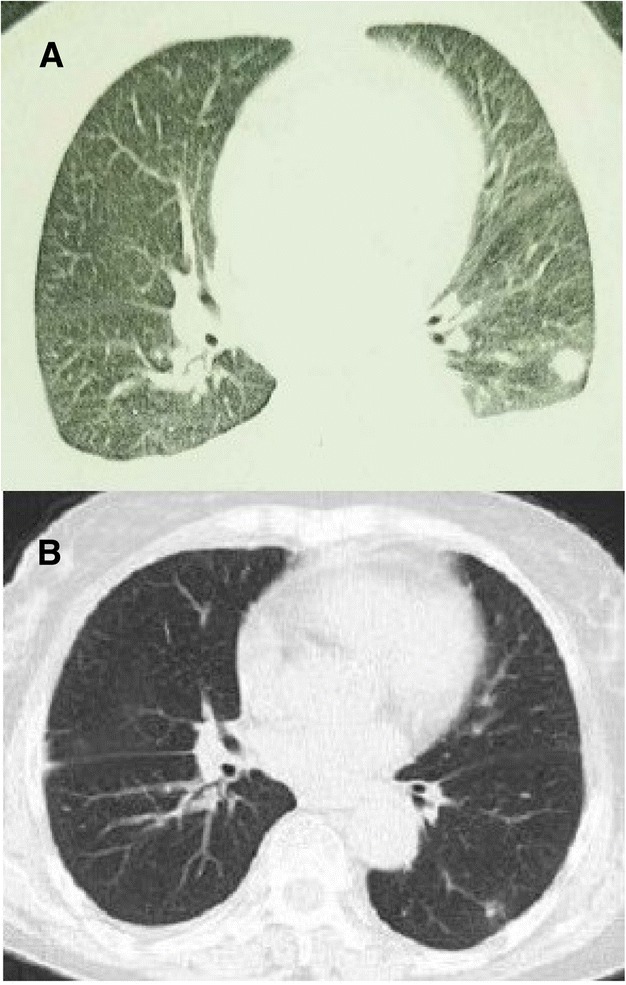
Chest CT scan in the axial plane performed 2 months following onset of symptoms showing (a) a nodule in the left lower lobe; the patient was administered fluconazole regularly for 4 months, and a repeated CT scan (b) indicated the absence of pulmonary nodule
